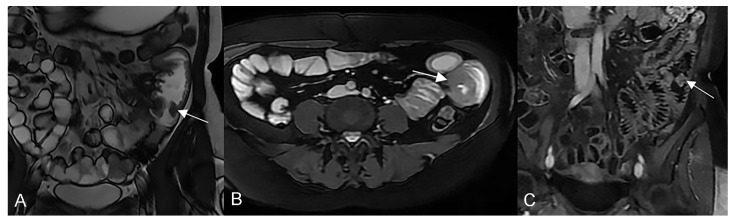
Example of adenocarcinoma in a middle-aged man. (A) Coronal T2-weighted, (B) axial T2-weighted fat-suppressed, and (C) coronal T1-weighted after gadolinium injection images show a focal irregular thickening of a jejunal loop (arrow) with inhomogeneous enhancement after gadolinium injection (arrow in (C)).
